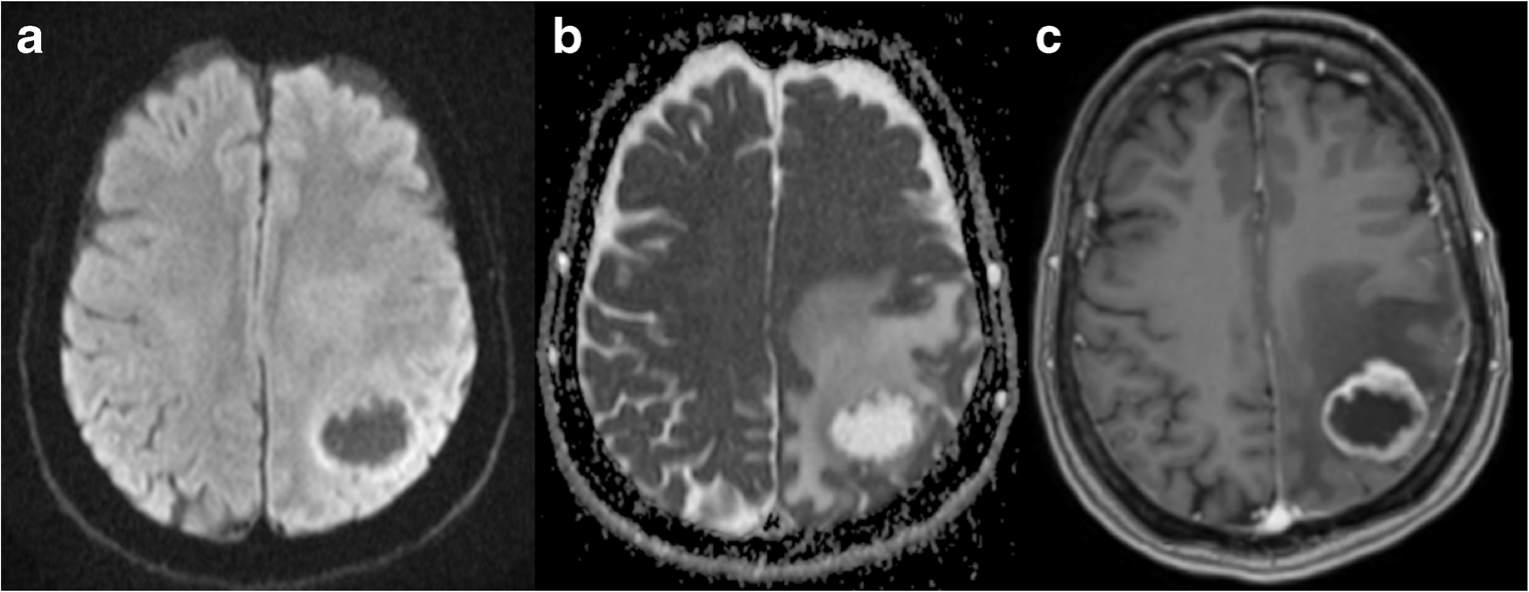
GBM. Left parieto-occipital lesion with peripheral vasogenic oedema. DWI and ADC (a and b, respectively) show a clear area of increased diffusion within the core, corresponding to a necrotic centre. On T1 3D post gadolinium the mass shows ring enhancement
